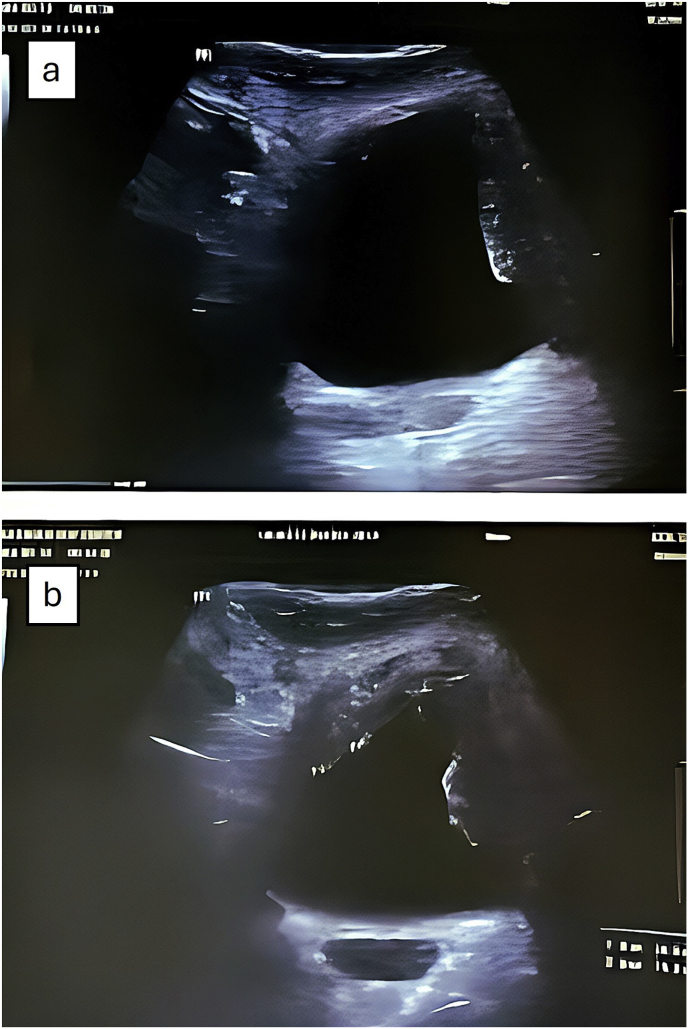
Ultrasonographic evaluation revealed irregular bladder wall thickening with multiple intraluminal projections which is shown in panel A and B in different view. The findings were initially interpreted as suggestive of multifocal bladder tumors.
